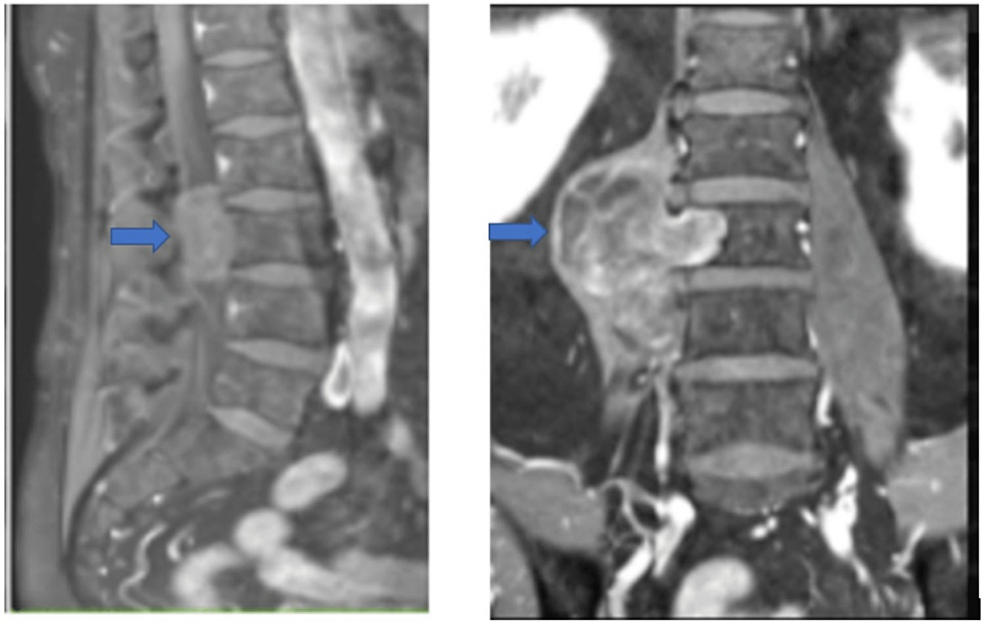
Preoperative surgical stage: (A) sagittal and (B) coronal lumbosacral MRI with contrast. The images show a well-defined mass at the L3-L4 level with compression in the right nerve root (blue arrow). MRI, magnetic resonance imaging
